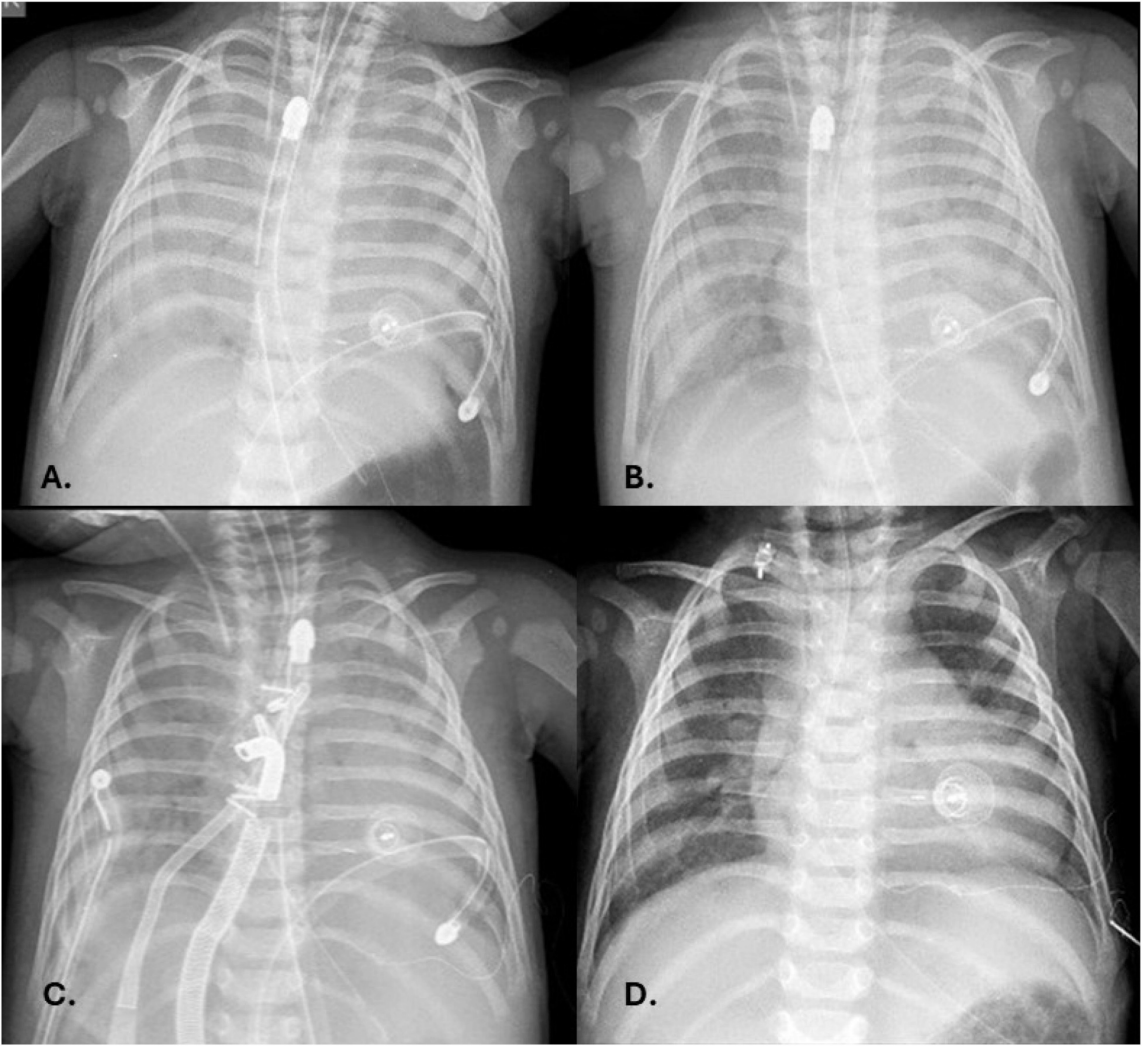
X-ray findings immediately after massive pulmonary haemorrhage (A); day after (B); after ECMO initiation (C); on discharge (D).
